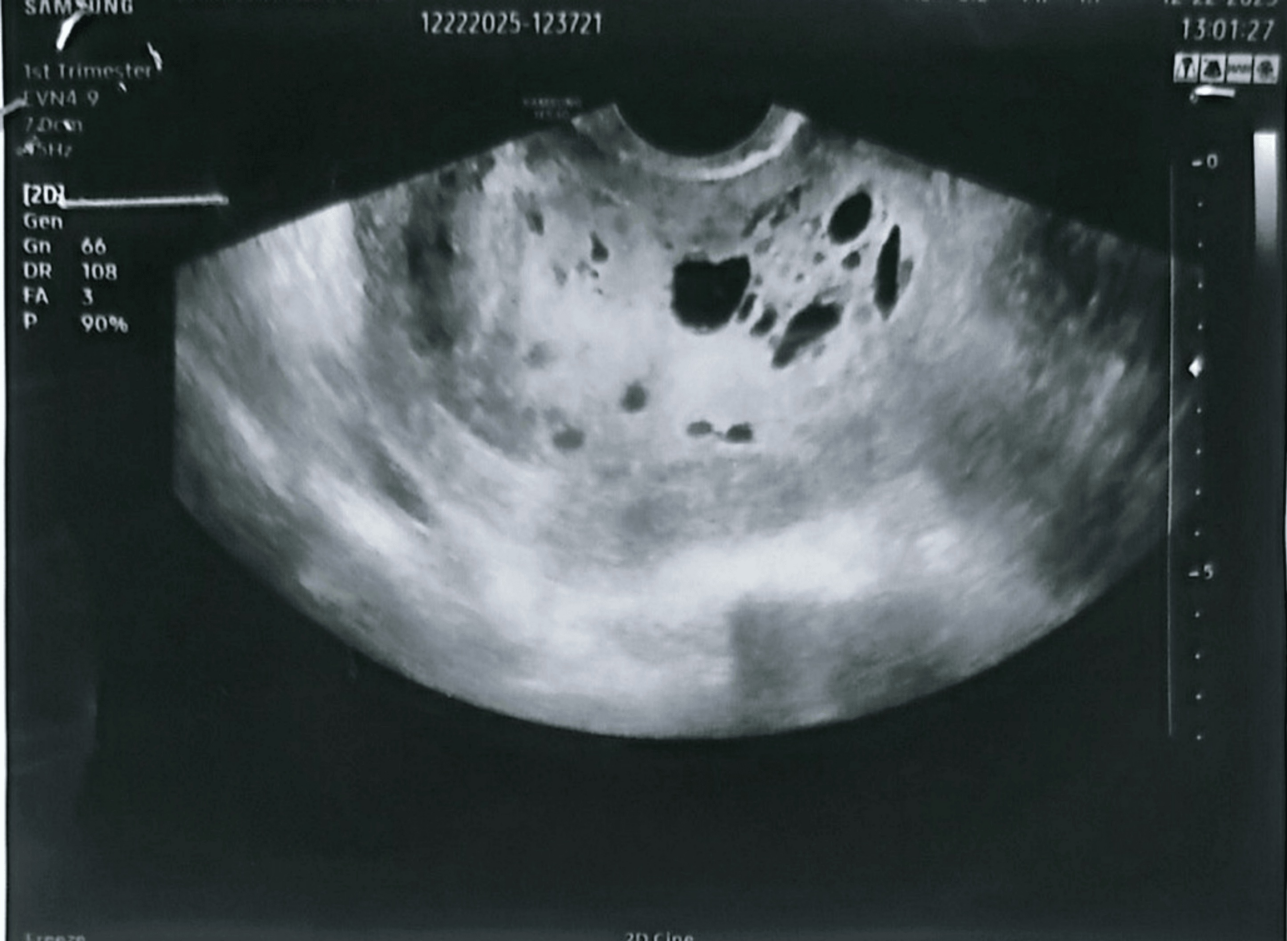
Transvaginal Ultrasound Demonstrating Diffuse Cystic Intrauterine Changes Without Identifiable Fetal Parts
